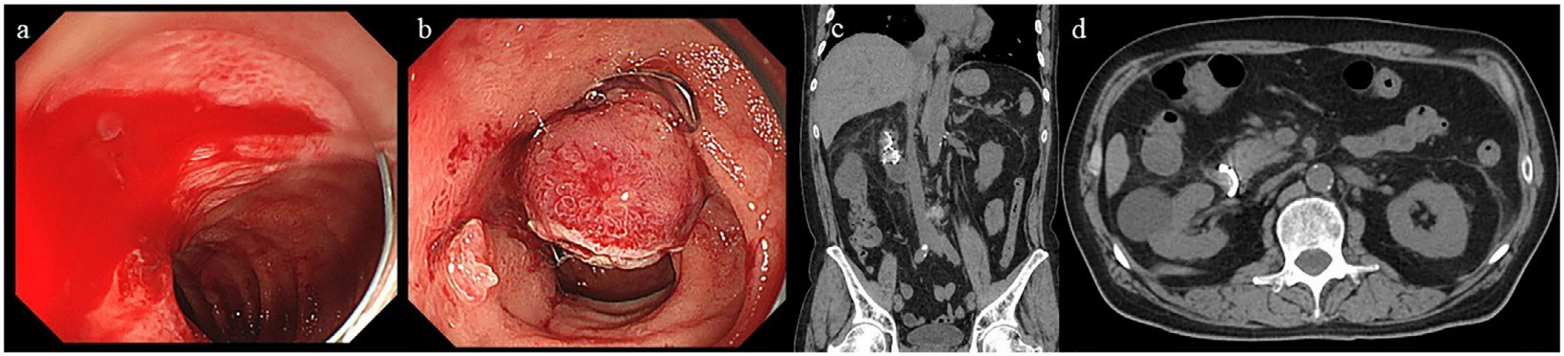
Case of successful hemostasis with over‐the‐scope clip (OTSC) followed by acute pancreatitis. (a) Endoscopic view showing a broad‐based ulcer with oozing hemorrhage in the horizontal section of the duodenum. (b) Two OTSCs were deployed, resulting in successful hemostasis. (c, d) A few days later, the patient developed mild acute pancreatitis. Abdominal computed tomography demonstrated increased fat stranding around the pancreas. (c) Coronal view; (d) axial view.
